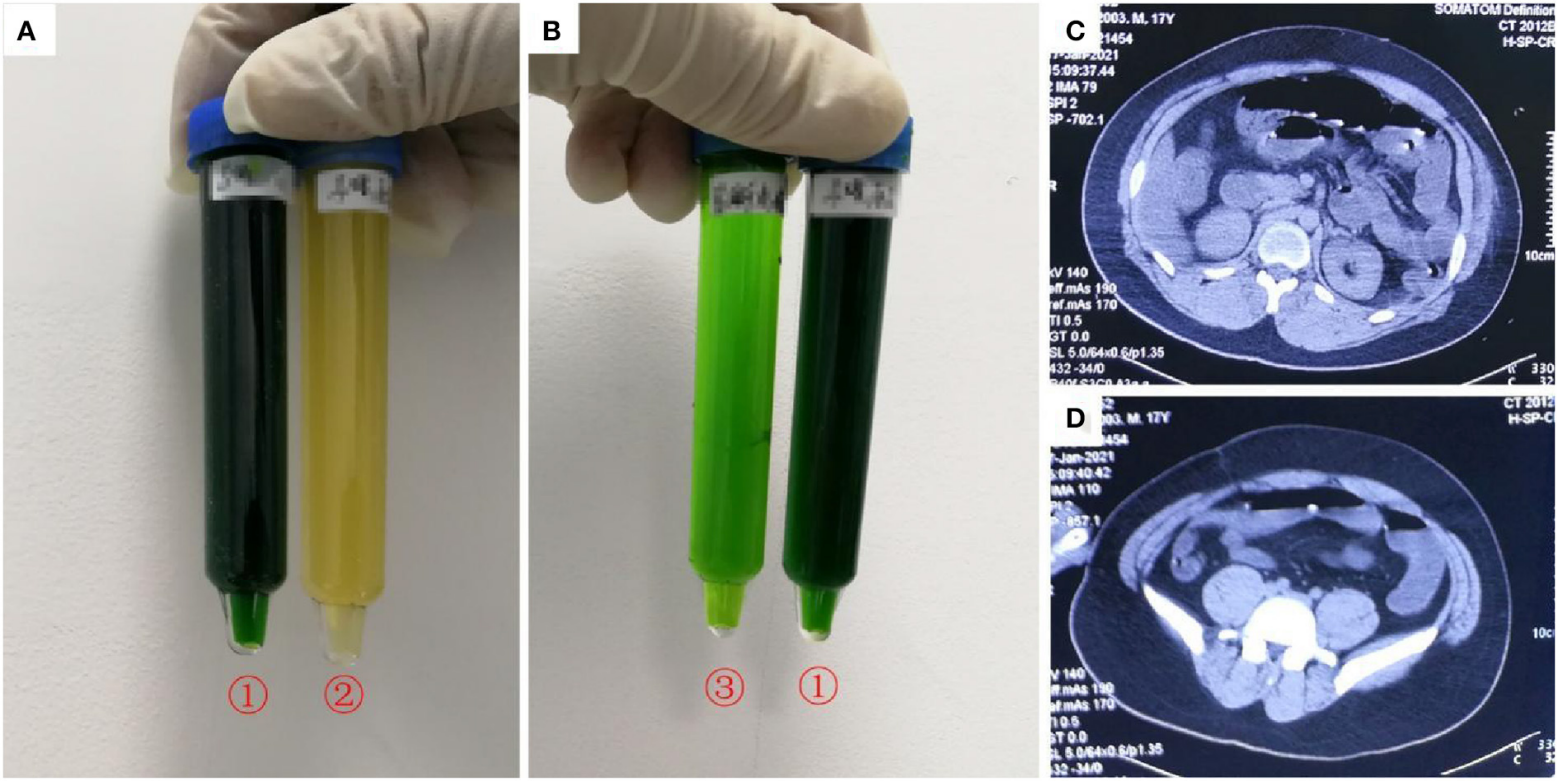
Images of urine and computerized tomography (CT) for the 21-year-old man who ingested ~200 mL of diquat. As shown in (A,B), after exposure to sodium dithionite, the patient's urine turned dark green (tube no. 1); the patient's original urine was darker in color (tube no. 2); the patient's urine turned light green after dilution (tube no. 3). (C,D) Shows partial intestinal gas and dilatation at the fluid level.
